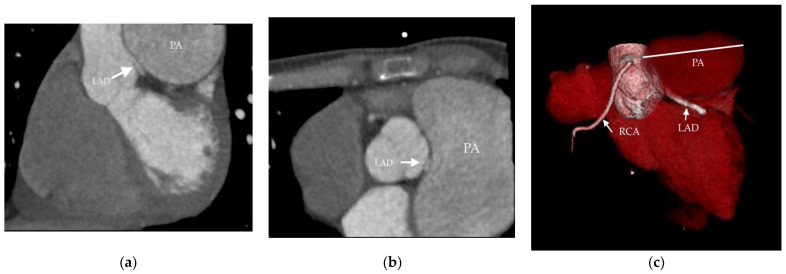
Coronary computed tomography angiography (Patient 1). The arrow shows the left ascending artery (LAD) compressed by dilated pulmonary artery (PA): (a) sagittal view; (b) axial view; (c) 3D reconstruction.
